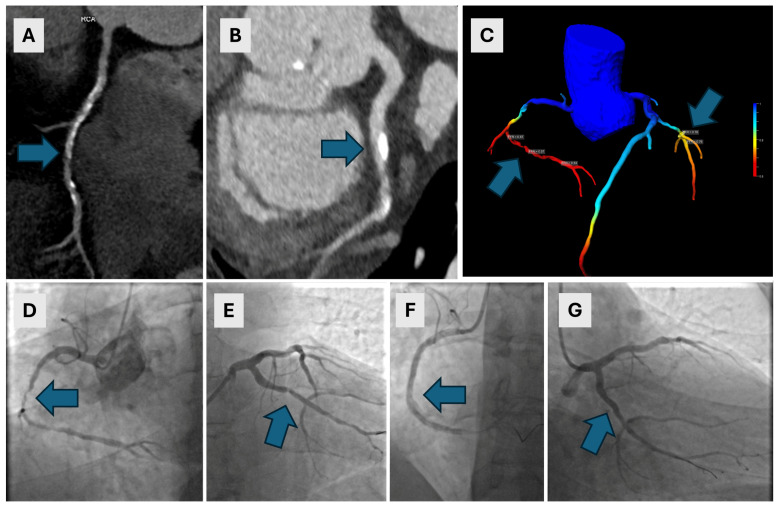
A 63-year-old male with a history of arterial hypertension and nonspecific arrhythmia underwent CCTA. The CAC score was 1406.7. Extensive calcified and mixed plaques were found in the RCA, with severe stenosis of up to 85% and in the RCA ((A)—arrow), and moderate stenosis of 65% in the LCX ((B)—arrow). A cFFR confirmed the significance of both lesions, with cFFR values of less than 0.7 in the RCA and 0.76 in the LCX ((C)—arrows). ICA revealed long 80–99% stenoses in the middle segment of the RCA ((D)—arrow) and a 90% stenosis in the proximal LCX ((E)—arrow). Immediate PCI of the RCA was performed with complete revascularization ((F)—arrow) which was complicated by anaphylactic shock. A few days later, after premedication, PCI of the LCX was performed with stent implantation and complete revascularization ((G)—arrow). CCTA—coronary computed tomographic angiography, CAC—coronary artery calcium, RCA—right coronary artery, LCX—left circumflex artery, cFFR—computed fractional flow reserve, ICA—invasive coronary angiography, PCI—percutaneous coronary intervention.
